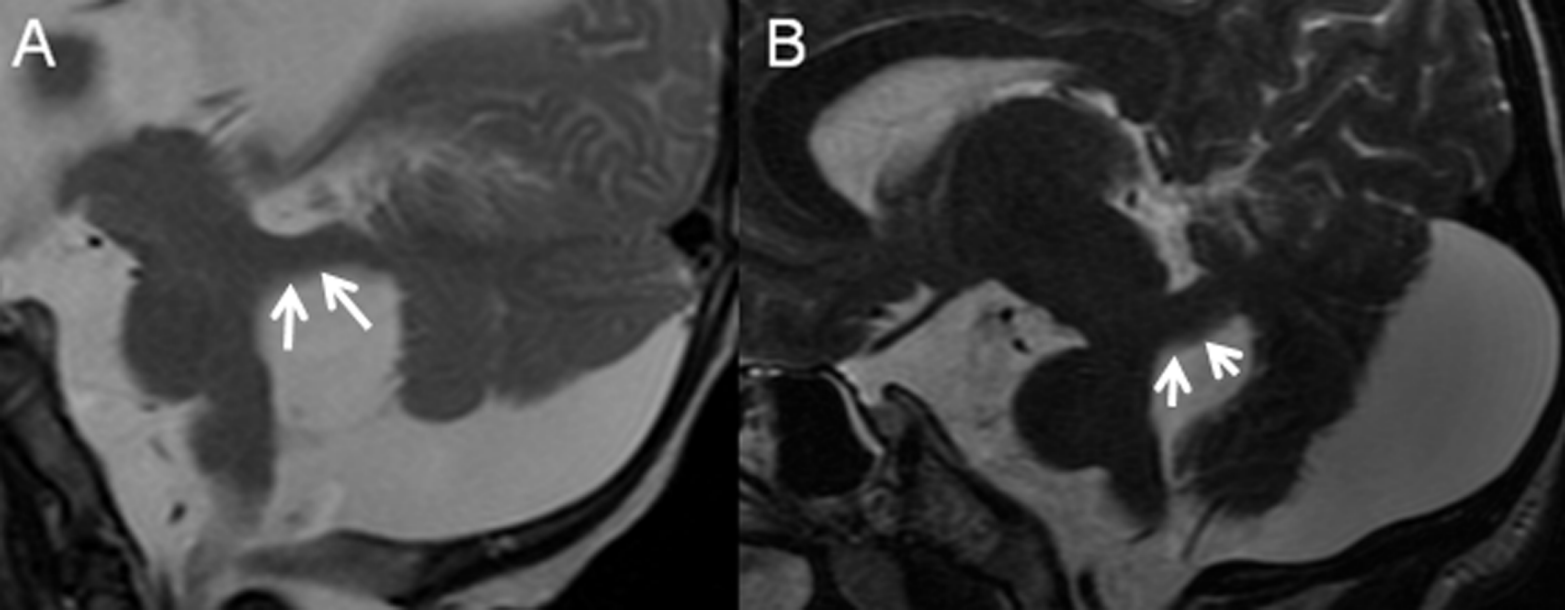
Parasagittal T2-weighted MR images of a 5-month-old infant (A) and a 22-year-old woman (B) with OFD VI. In JSRD the SCP (white arrows) have a characteristic horizontal sagittal orientation (A). In some patients with OFD VI, however, the SCP (white arrows) have a clearly ascending orientation (B).
